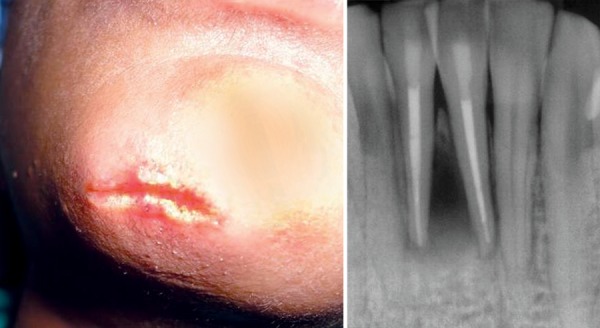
Postoperative photograph and radiograph after 1 month
